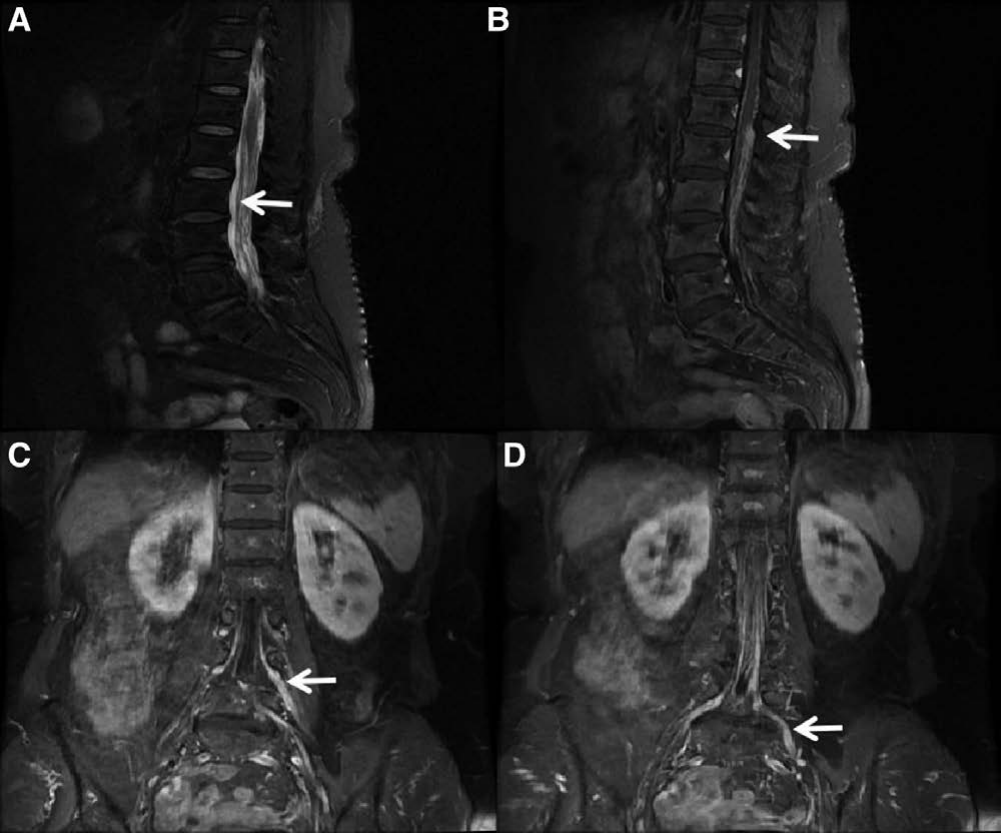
Imaging. (A) Contrast lumbar spine MRI demonstrates multiple abnormal enhancements in the spinal canal of the lower part of the thoracic vertebrae in the 10-lumbosacral region, mainly within the dura mater and terminal filaments. (B) The lumbar 1–2 intervertebral space level of the right spinal has nodule-like enhancement, the border of which is not vague, and the range is approximately 0.4 cm × 0.6 cm × 1.0 cm. (C&D) Enhancement can be seen along the nerve root in the lumbar 4- sacral 1 intervertebral foramen. MRI = magnetic resonance imaging.
